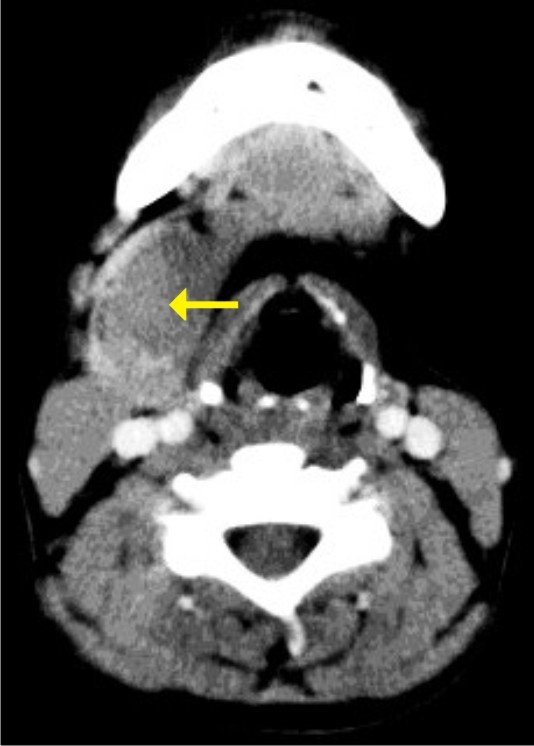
Computed tomographic scan showing a minimally enhancing hypodense lesion (arrow) within the right submandibular salivary gland with an enhancing rim of the normal submandibular gland at the periphery.
